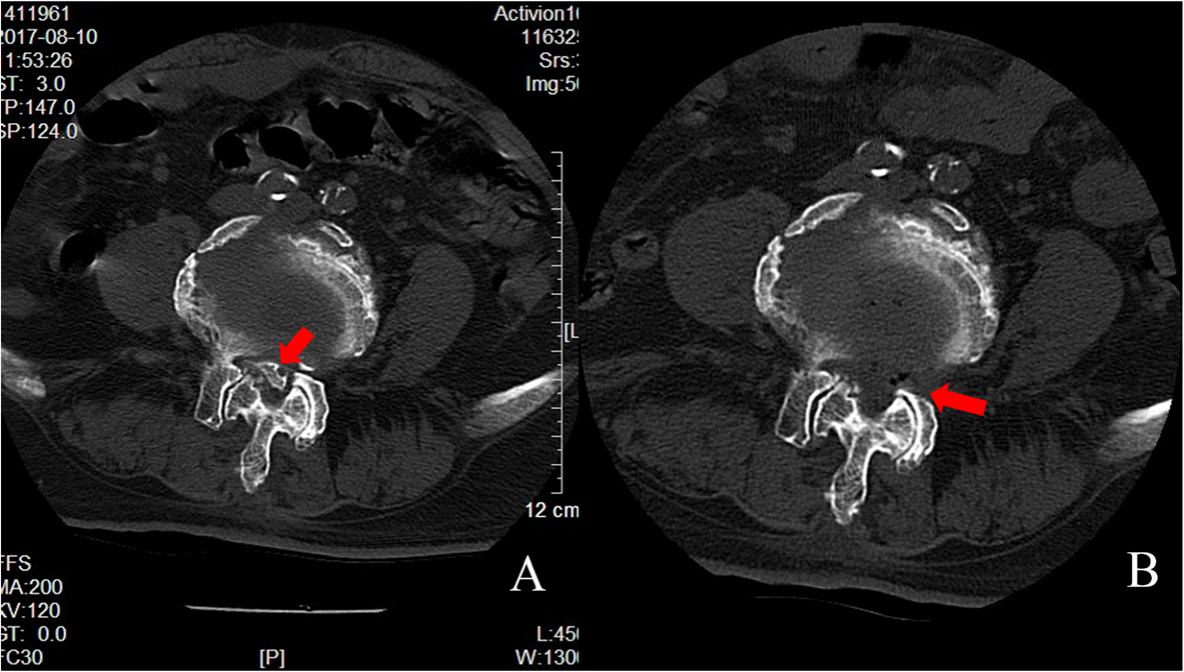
CT image showed (a) L4/L5 lumbar spinal stenosis and calcified lumbar disc herniation (b) Lumbar foraminoplasty (left) and decompression with removal of the calcified lumbar disc herniation (indicated by red arrows)
